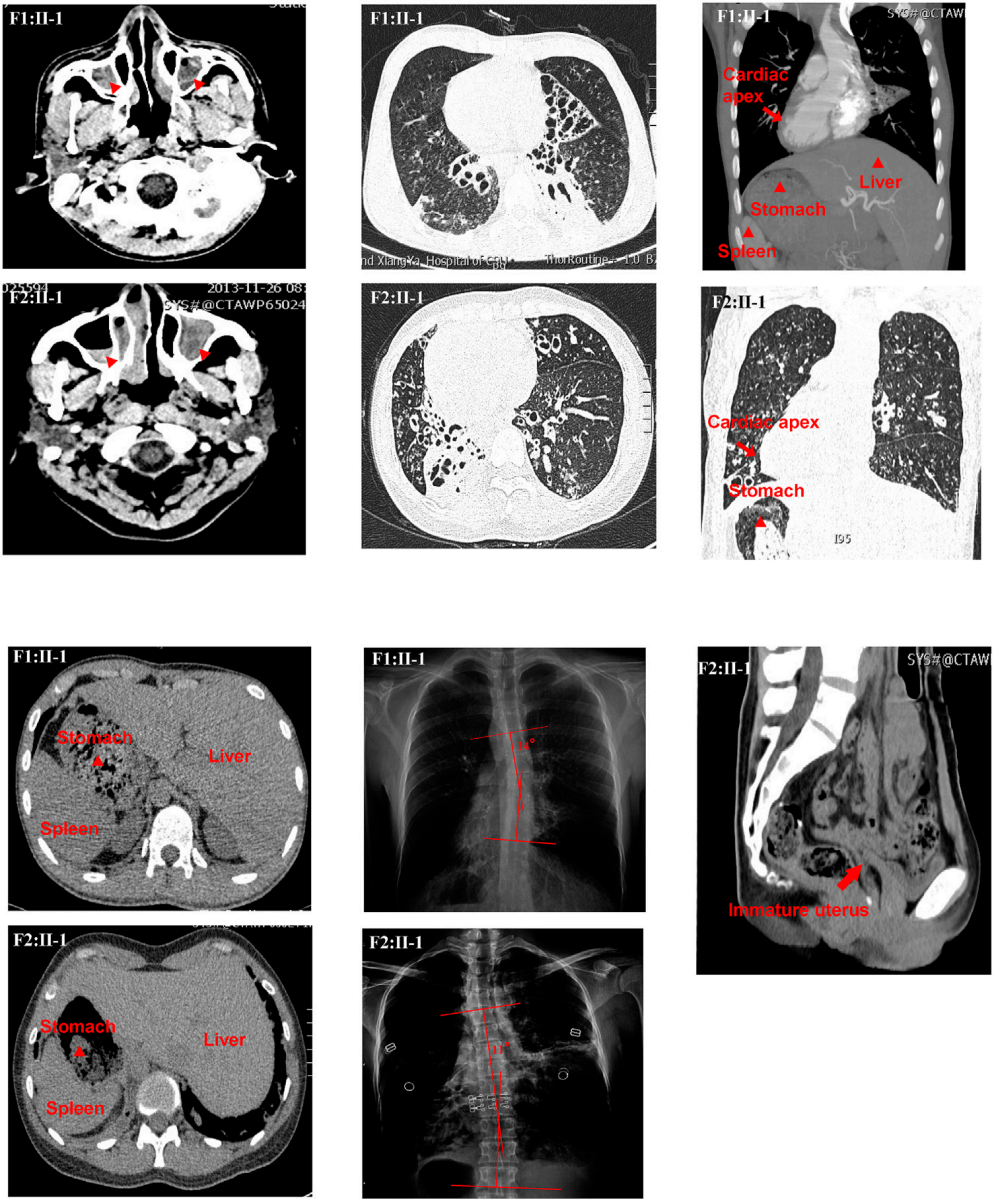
Clinical features of the two patients. The chest high-resolution computed tomography (HRCT) scan of P1 (F1:Ⅱ-1) showed bronchiectasis, rhinosinusitis, and situs inversus. Radiographic image of P1 (F1:Ⅱ-1) showed scoliosis with a Cobb’s angle of 14°. The HRCT scan of P2 (F2:Ⅱ-1) showed bronchiectasis, rhinosinusitis, situs inversus, and immature uterus. Radiographic image of P2 (F2:Ⅱ-1) showed scoliosis with a Cobb’s angle of 11°.
